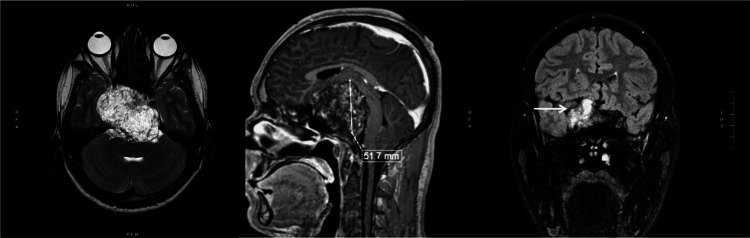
Brain MRI: (1) extreme left is T2-weighted axial fat saturation image showing a large intracranial tumor in the region of the clivus; (2) Middle image is post contrast T1-weighted sagittal view showing the large (51.7 mm height) tumor displacing superiorly the midbrain and the chiasm region; (3) Extreme right is T2-weighted coronal FLAIR image showing the tumor (arrow) extending to the right side. FLAIR: Fluid-attenuated inversion recovery
